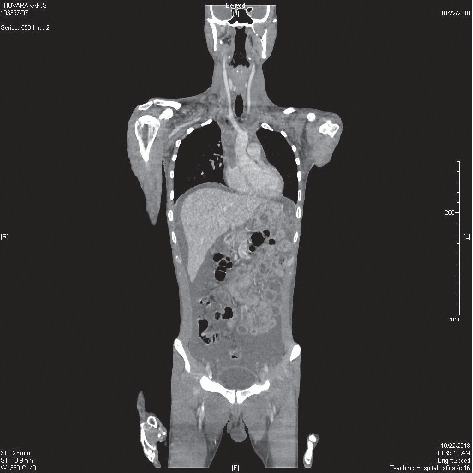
The contrast-enhanced computed tomography of neck, chest, abdomen, and pelvis showed extensive venous thrombosis involving in internal jugular veins, subclavian veins, brachiocephalic vein, and superior vena cava up to the right atrium.
